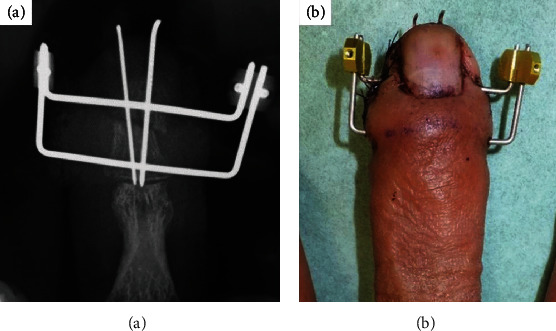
Postoperative radiographic (a) and clinical views (b).
